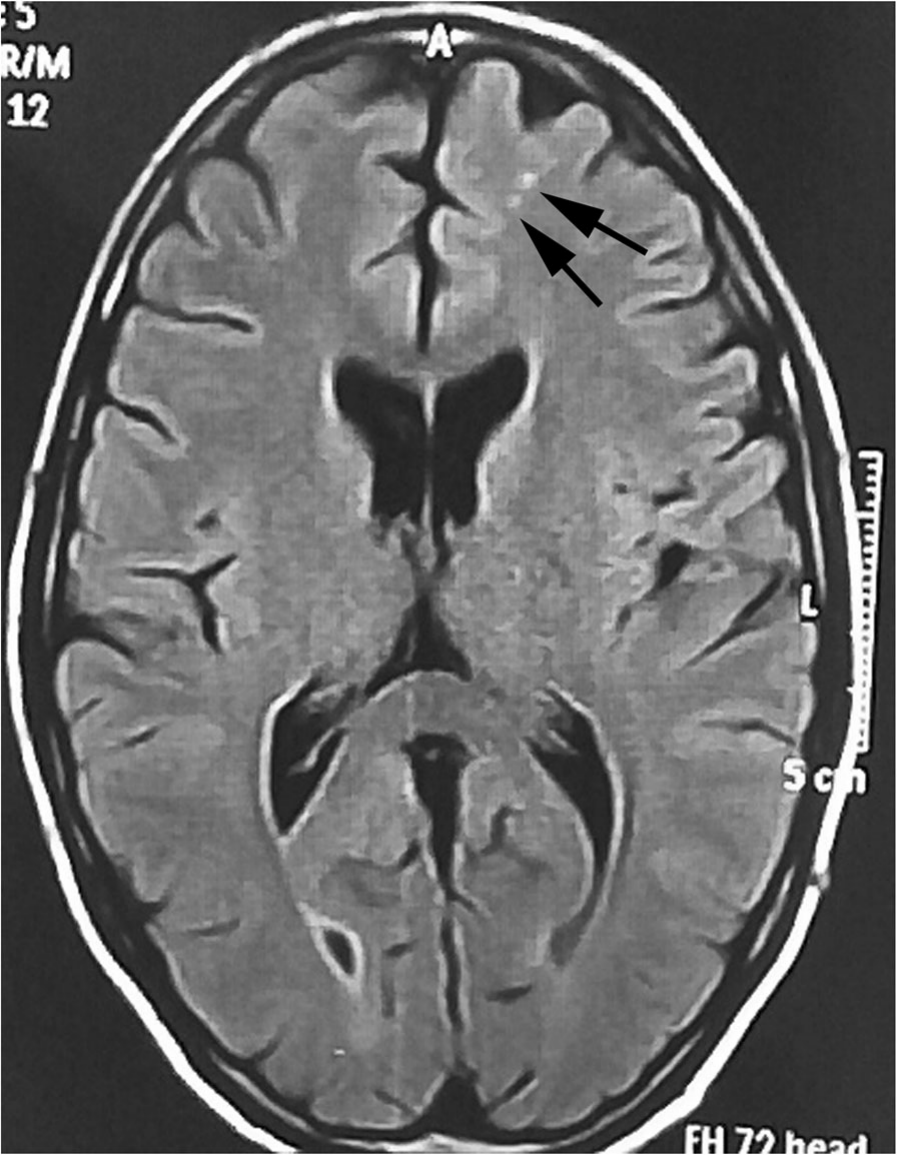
25-year-old female patient, not known to have any chronic illness, presented with migraine without aura for 6-year duration of grade II severity, not responding to medical treatment. Axial FLAIR MRI image shows two left frontal white matter hyperintense lesions (arrows)
